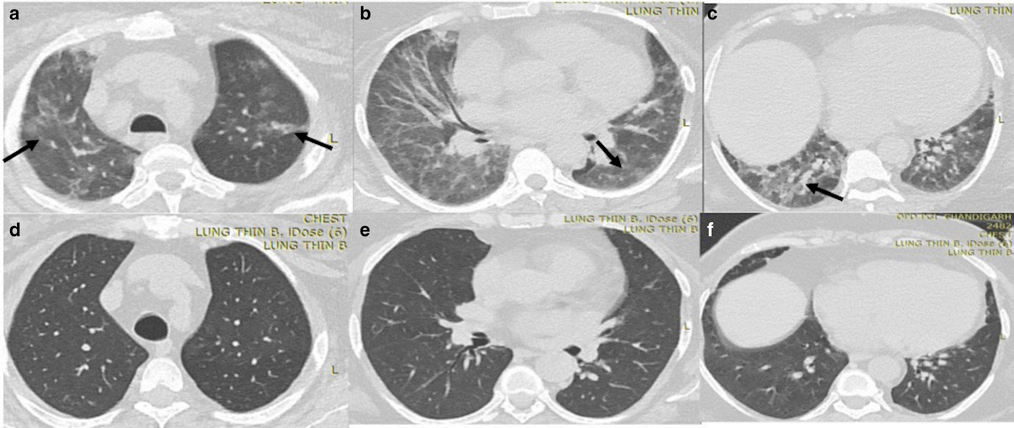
CT chest images of a 40-year-old female. (a–c): Axial
